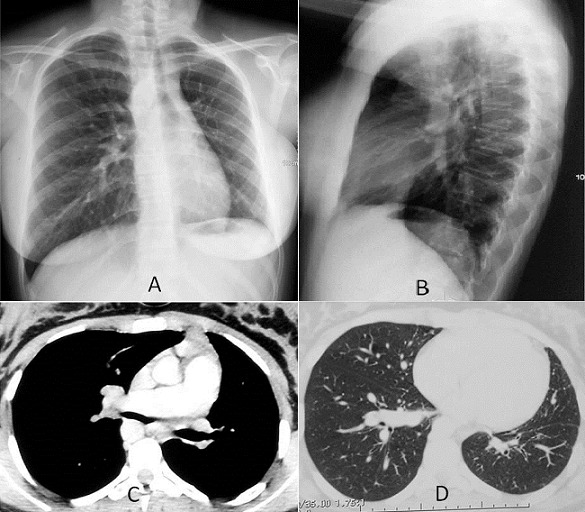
(A, B) radiographie thoracique de face et de profil montrant un petit champ pulmonaire gauche avec déviation médiastinale gauche; C) fenêtre médiastinale de l’angioscanner thoracique objectivant l’absence de l’artère pulmonaire gauche et l’aorte descendante placée à droite; D) fenêtre parenchymateuse du scanner thoracique montrant un poumon gauche hypoplasique mais bien aéré
